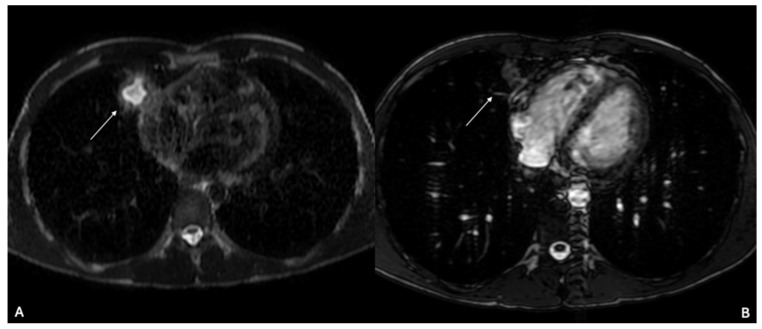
Axial T2-weighted fast spin-echo (FSE) image (A) shows a complex pulmonary lesion in the left lung (arrow) with marked T2 hyperintensity, consistent with a necrotic–liquefactive component complicating underlying lipoid pneumonia. Axial balanced steady-state free precession sequence (FIESTA) (B) (arrow) better delineates the lesion contours and its relationships with adjacent mediastinal structures, aiding pre-procedural/surgical planning and assessment for mediastinal contact or involvement.
